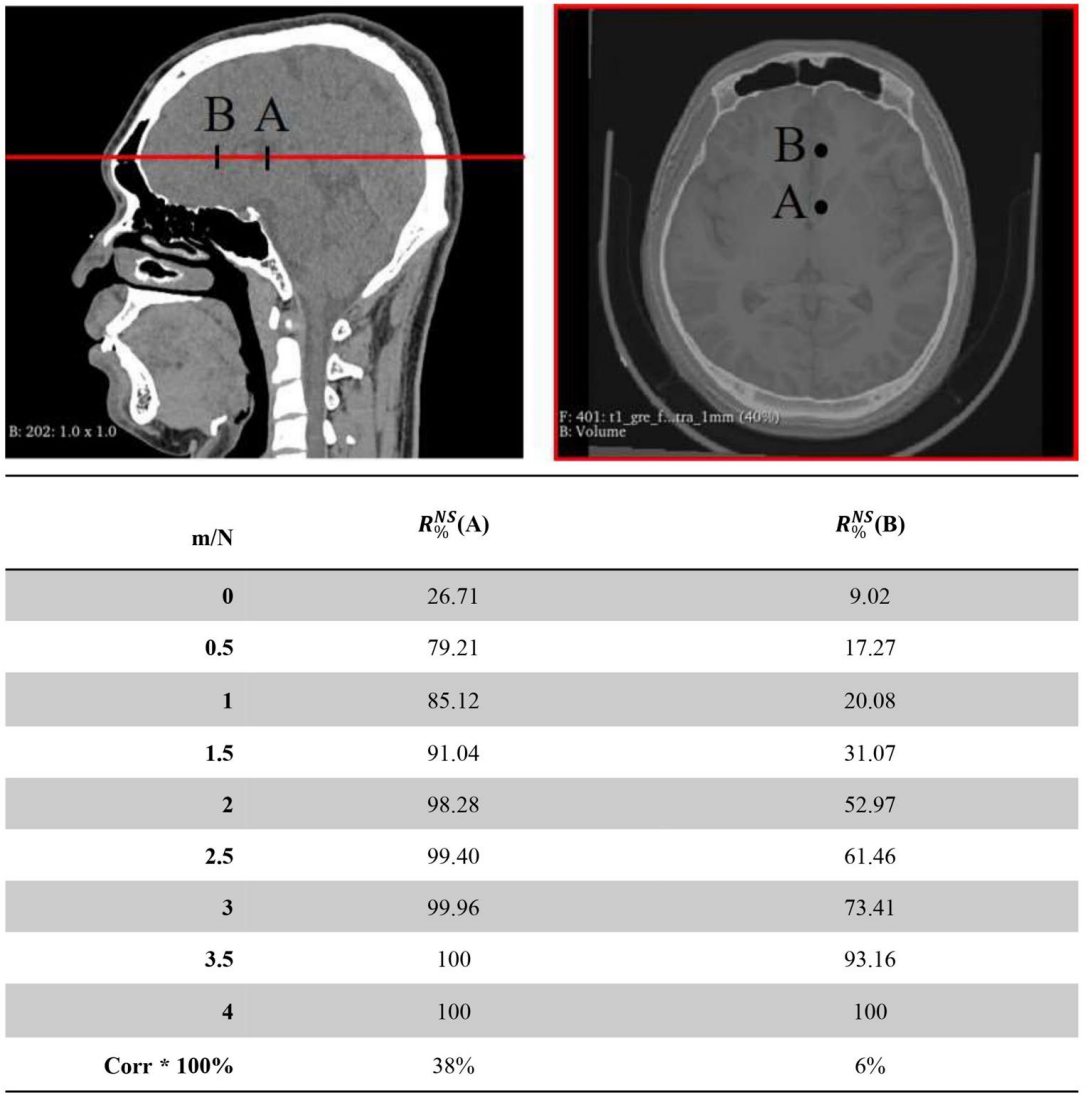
The focusing performance at different target points, with A virtually around the ventral intermediate nucleus (VIM), and B virtually around the corpus callosum, was evaluated. The CT and fusion image data used in this figure originate directly from the subject.
